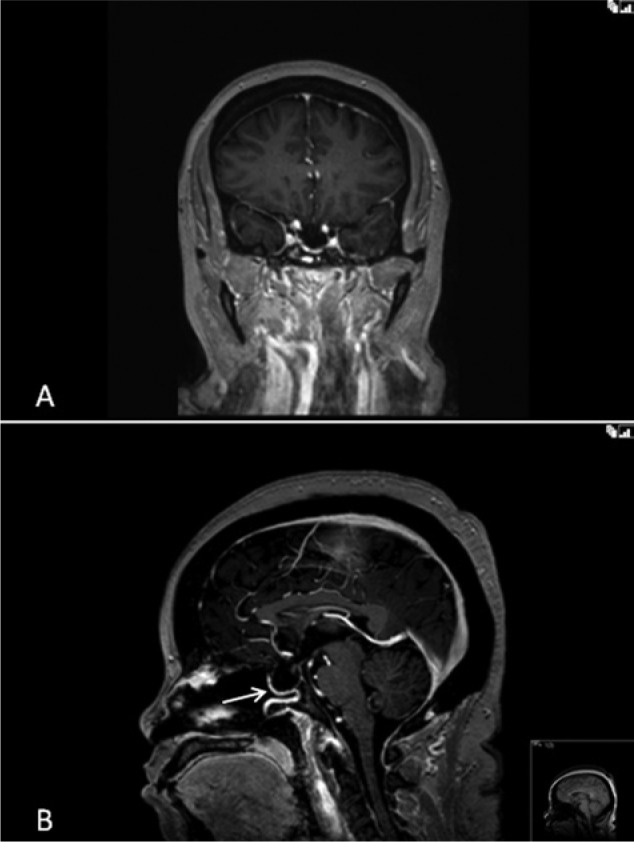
Coronal (A) and sagittal (B) post-contrast magnetic resonance images 16 months after the cerebrospinal fluid (CSF) leak repair show resolution of the dural enhancement and subdural hygromas seen in Figure 1 (C-D). Note the presence of a vascularized septal flap (arrow, B) and return of the brainstem and cerebellum to a normal anatomic position in comparison to the inferior displacement noted in Figure 1 (D). These findings indicate full resolution of intracranial hypotension caused by the CSF leak.
